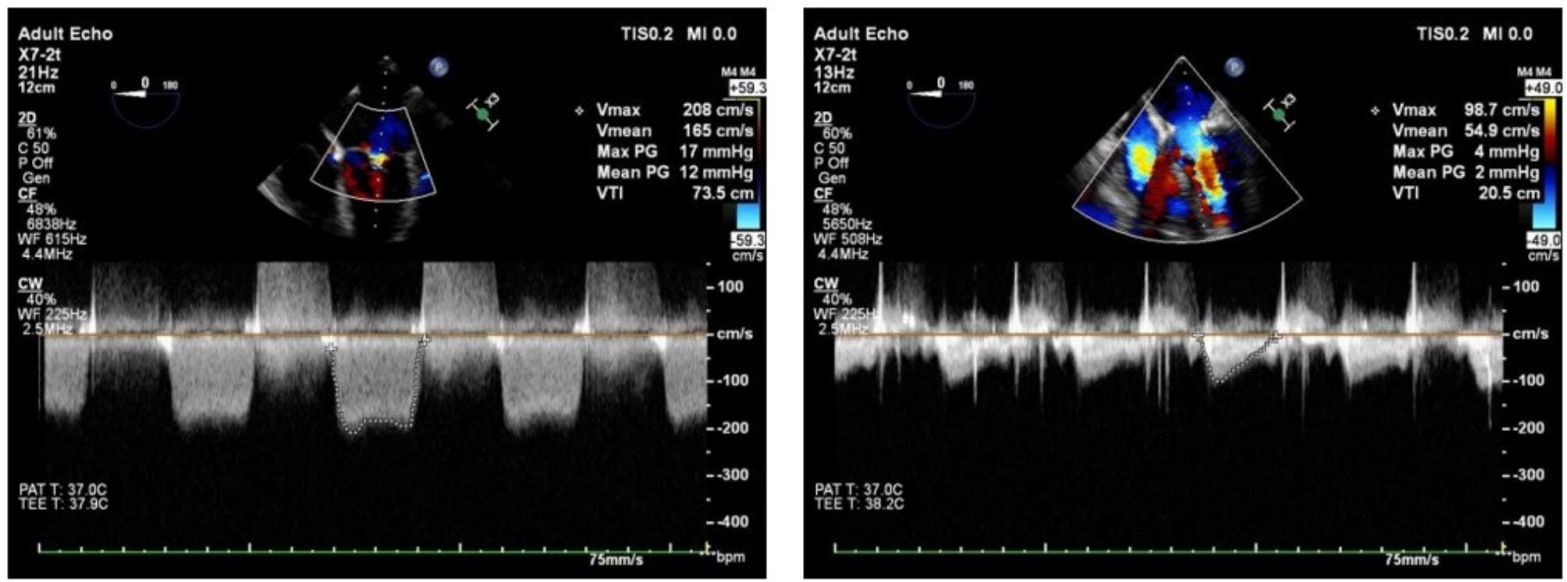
Intraoperative transesophageal ultrasound. (Left) Pre-MVP, end-diastolic peak flow velocity is 2 m/s, and Transvalvular pressure gradients are 17 mmHg. (Right) Post-MVP, End diastolic peak flow velocity 1 m/s, and Transvalvular pressure gradient is reduced to 4 mmHg
